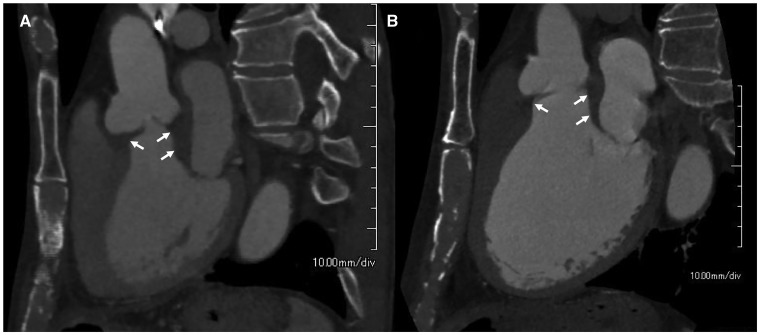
(*A*) Contrast-enhanced computed tomography at initial diagnosis showing the thickened aortic valve extending to the left ventricular outflow tract wall. (*B*) The preoperative contrast-enhanced computed tomography showing regression of the thickened aortic valve and left ventricular outflow tract wall.
